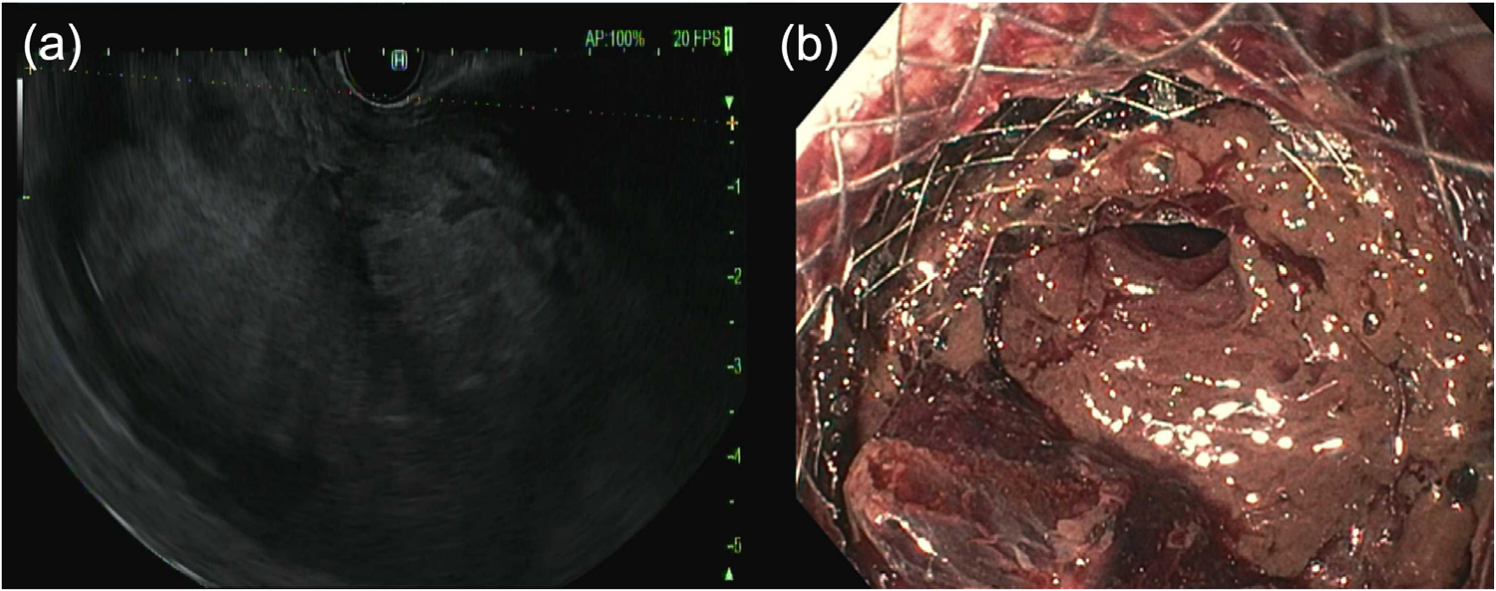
(a) Endoscopic ultrasonography showed evidence of a large pseudocyst with extensive hyperechoic material. (b) Lumen‐apposing metal stent was deployed by cystogastrostomy to reveal hemorrhagic necrotic material inside the pseudocyst.
